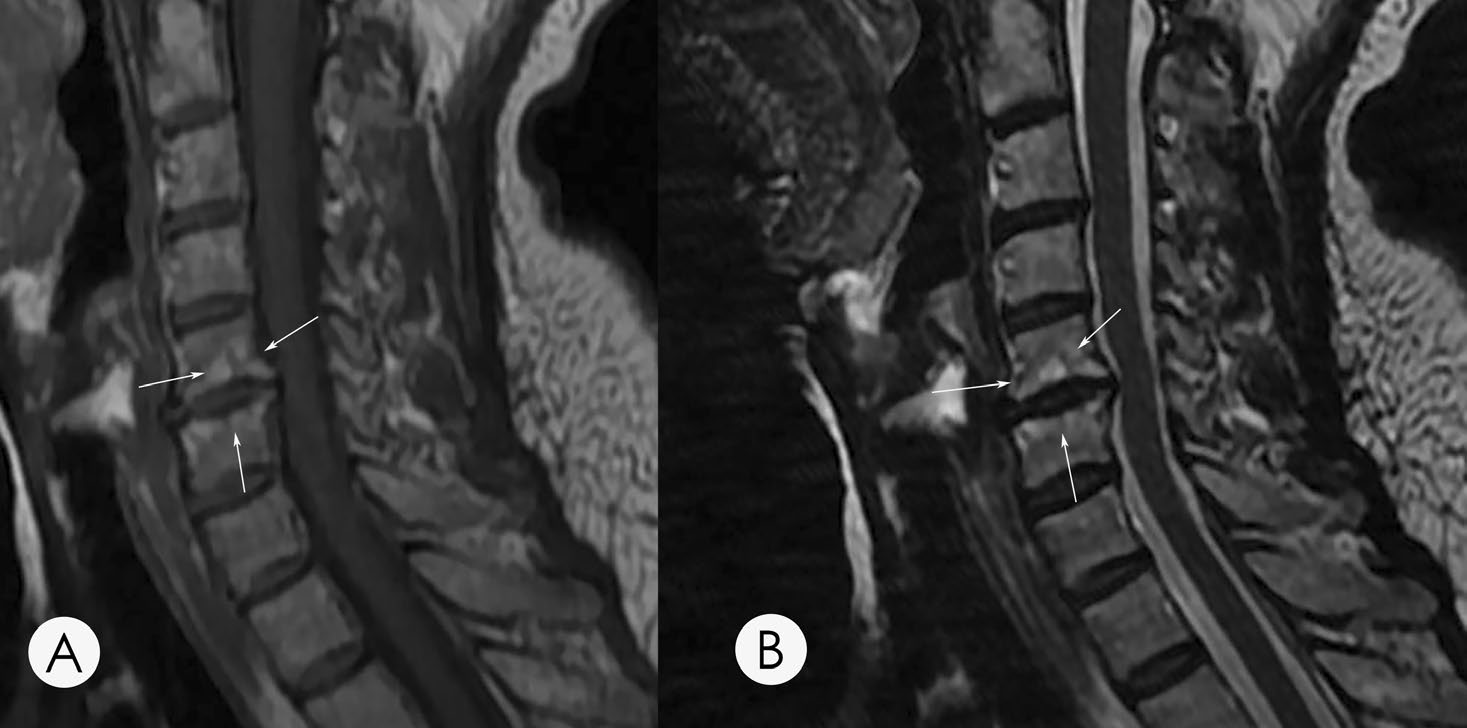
(A) A T1‐weighted magnetic resonance imaging (MRI) of a 48‐year‐old female shows hyperintensity at the inferior endplate of C4 and superior endplate of C5. (B) A T2‐weighted MRI of the same patients demonstrates hyperintensity, suggesting a type 2 MC. Arrows indicate the location of the identified MC lesion on T1 and T2 imaging.
